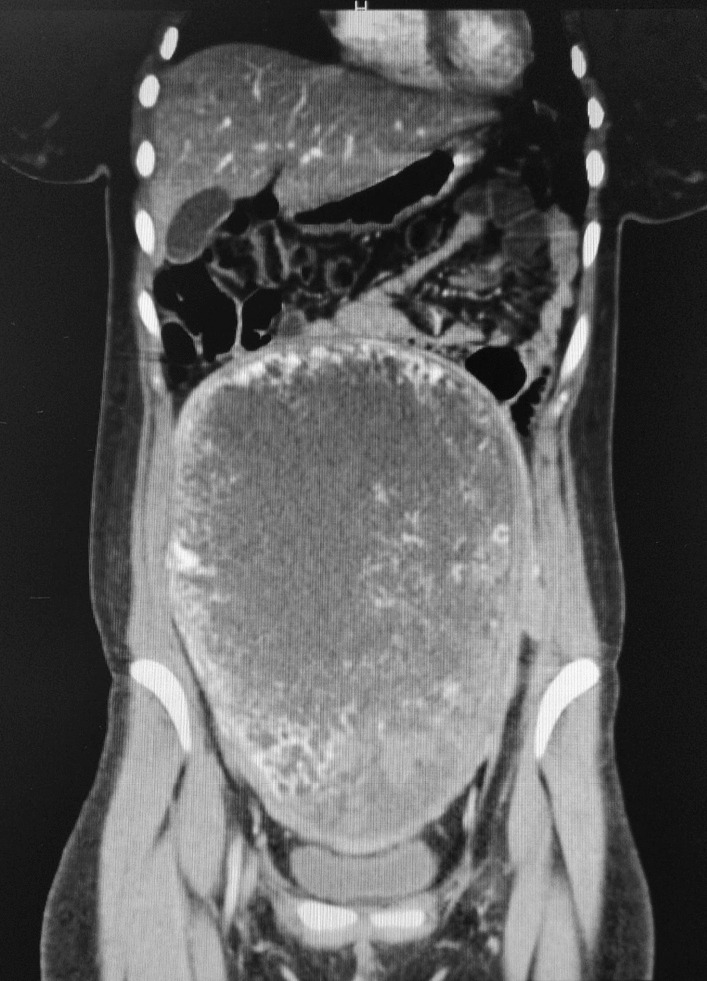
Preoperative thoracoabdominal CT scan
